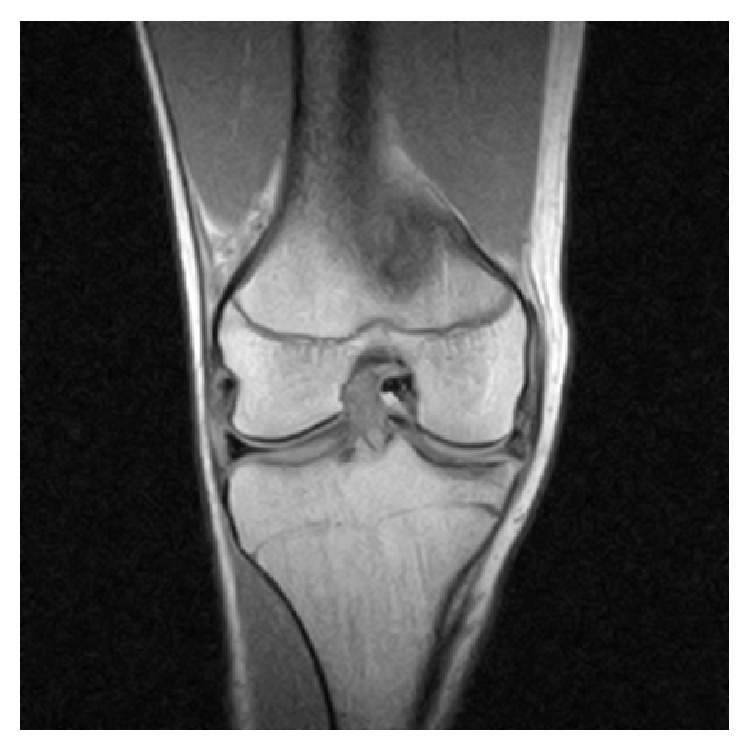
Coronal MRI of the right knee revealing intra-articular and bucket-handle medial meniscus tear with the displaced fragment located in the intercondylar notch.
